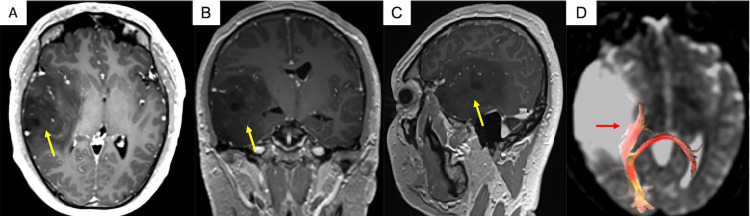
Contrast-enhanced MRI and DTI tractography. Contrast-enhanced MRI: (A) axial, (B) coronal, and (C) sagittal reconstructions in which a homogeneous, non-enhancing right temporal lesion is observed (yellow arrows). (D) DTI tractography showing displacement of the right visual pathways (red arrow). DTI, diffusion tensor imaging
